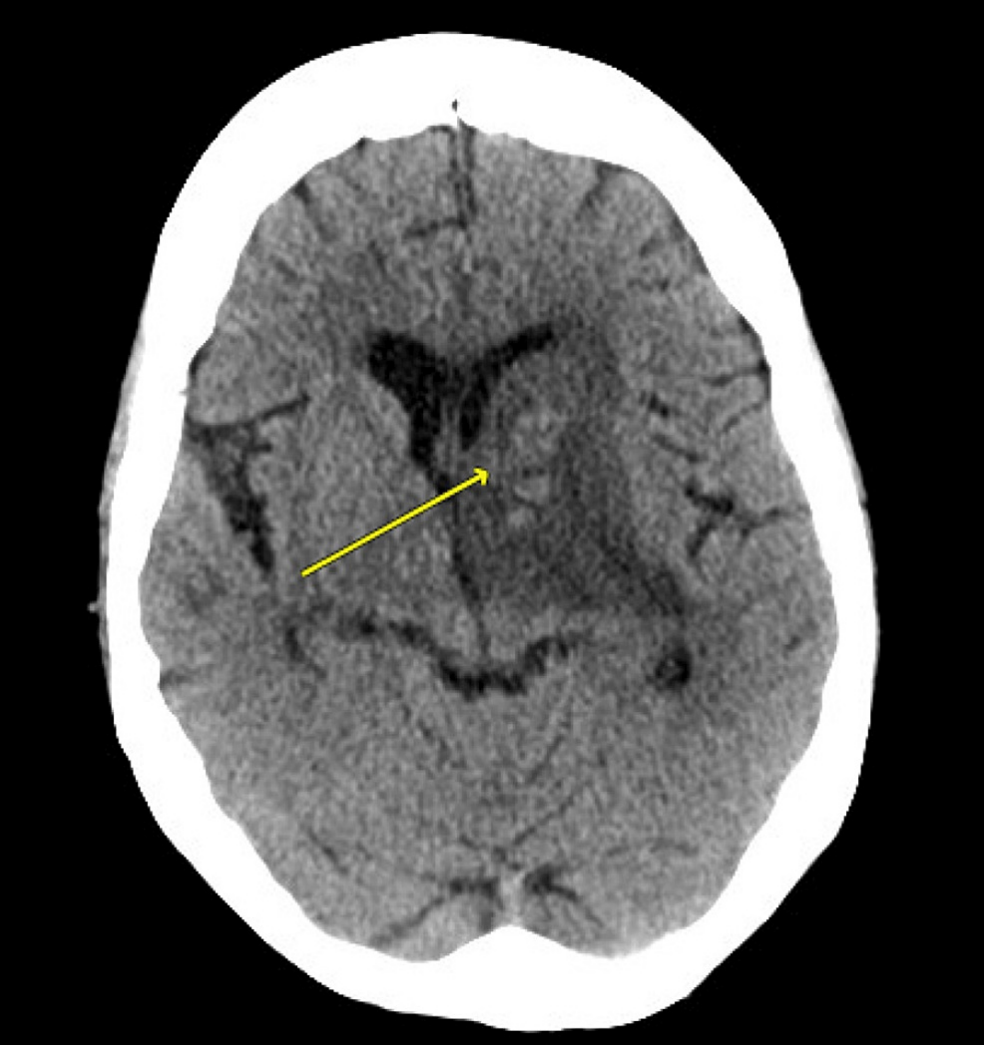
An axial image from the unenhanced CT scan of the head reveals significant central cerebral edema affecting the left basal ganglia and thalamus CT: computed tomography
